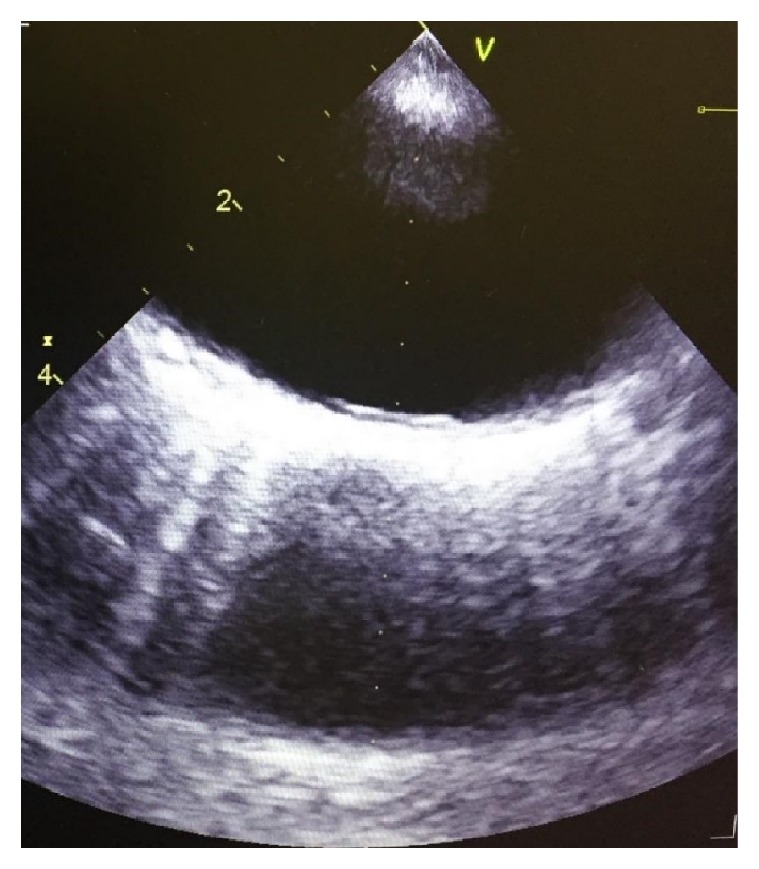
TEE demonstrating an echogenic density in the aortic arch, consistent with thrombus.* Abbreviation.* TEE: transesophageal echocardiogram.
